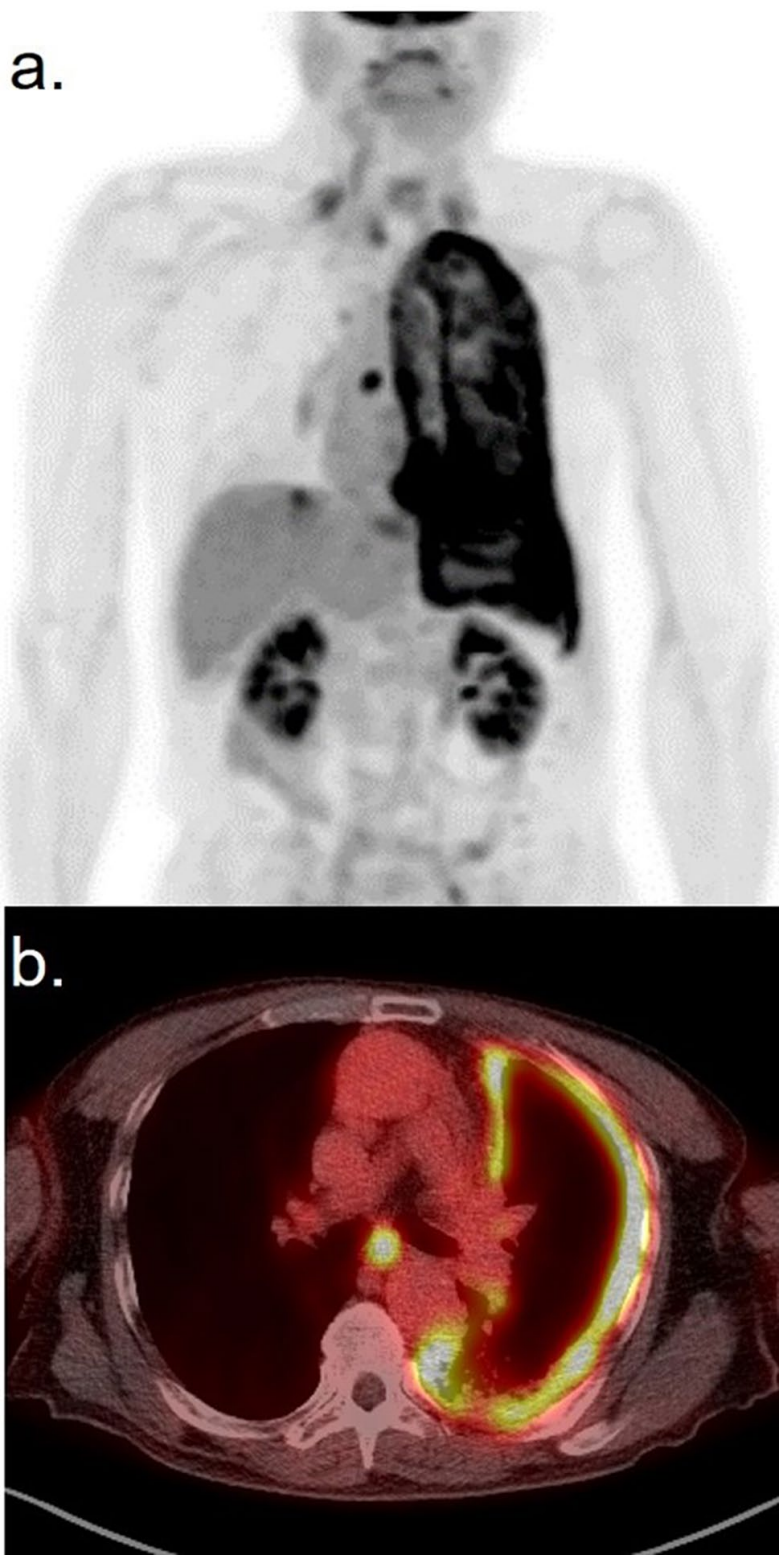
Intense FDG accumulation spreading inside the left pleura 1 month after pleurodesis with OK-432 after bruising the chest for pleuritis carcinomatosis. a MIP, b axial fusion image)
